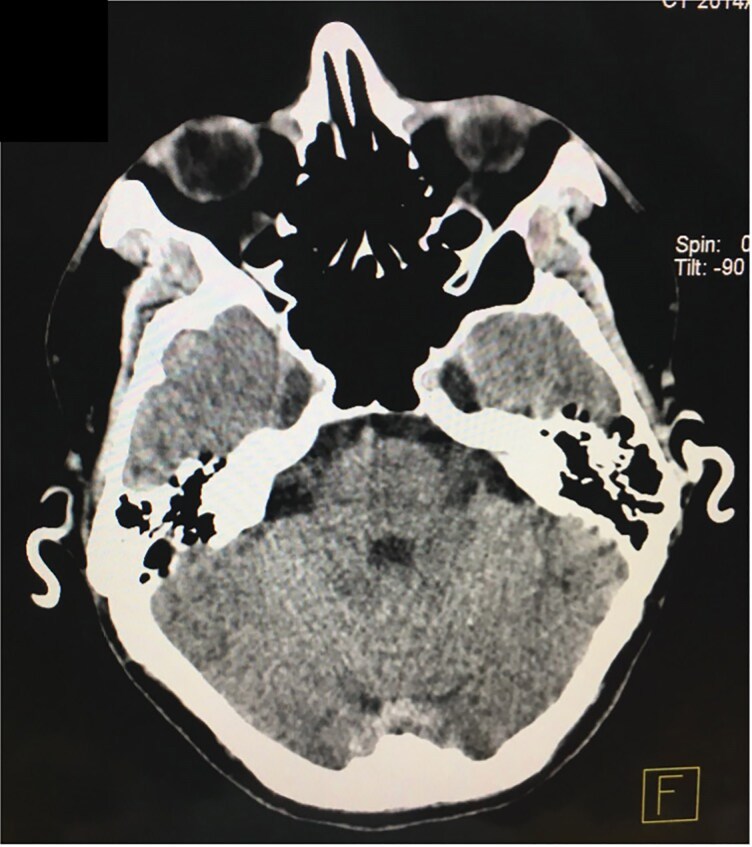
Axial computed tomography image showing bilateral auricular cartilage calcification.
